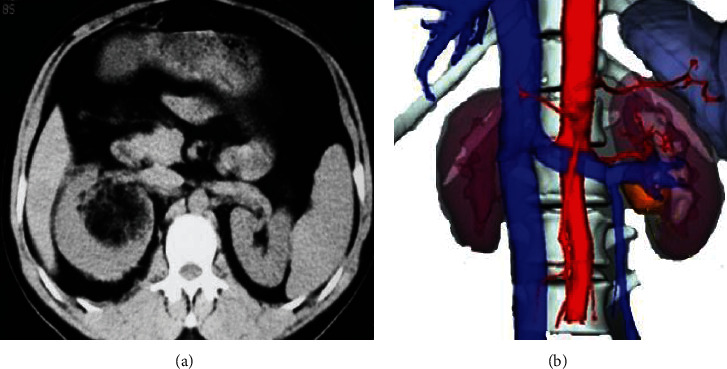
Images of renal tumor (male patient aged 48 years; the tumor was in the right kidney with the size of 4.7 cm). (a) 2D CT image of renal tumor. (b) CT image of renal tumor based on the 3D reconstruction algorithm.
